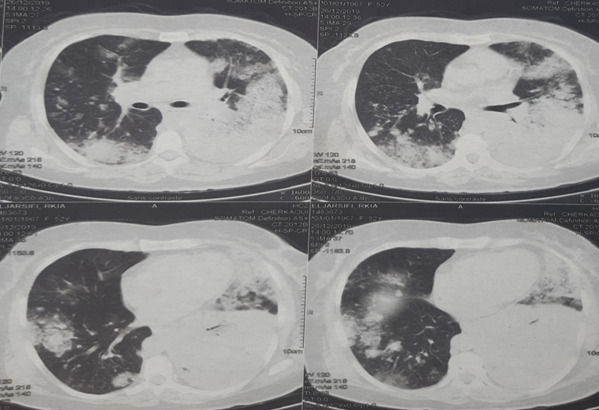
The thoracic computed tomography (CT) showing an alveolar condensation of the left lower lobe containing an air bronchogram, associated with multiple nodular lesions and alveolar condensation of the right lung
